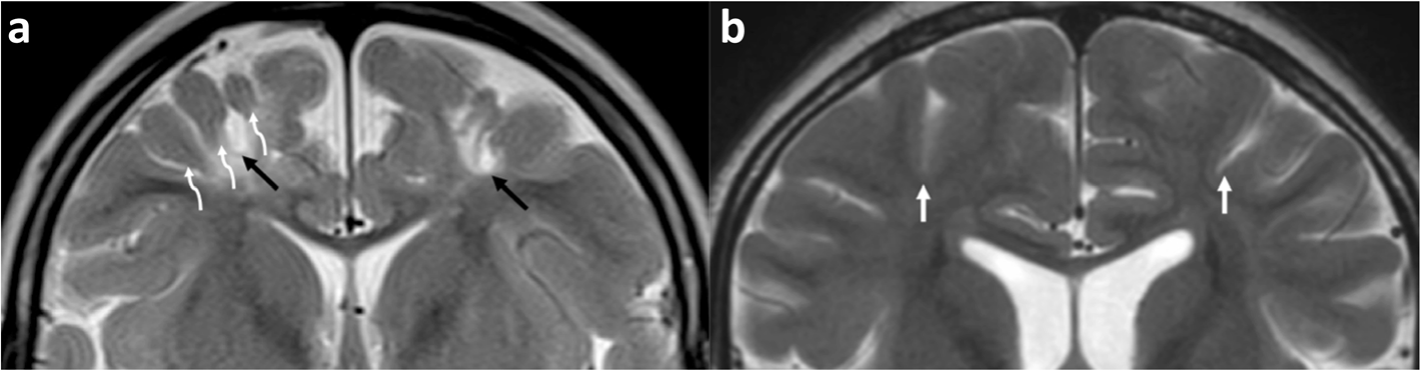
a, b Ulegyria in a patient with partial-prolonged HII in prolonged labour (a) compared with normal gyral and sulcal pattern (b). Coronal T2-weighted image in a, a 4-year-old boy, who suffered a partial-prolonged HII demonstrating bilateral, symmetric localised atrophy in the parasagittal regions and abnormal underlying white matter high signal. There is ulegyria with overt thinning of the stems (curved white arrows) of the involved gyri and a resultant teardrop shape to the sulcus (black arrows). In comparison, the gyri in b, a normal 8-year-old girl, have parallel walls throughout their length and the sulci remain slit like from their surface to their depth (straight white arrows)
